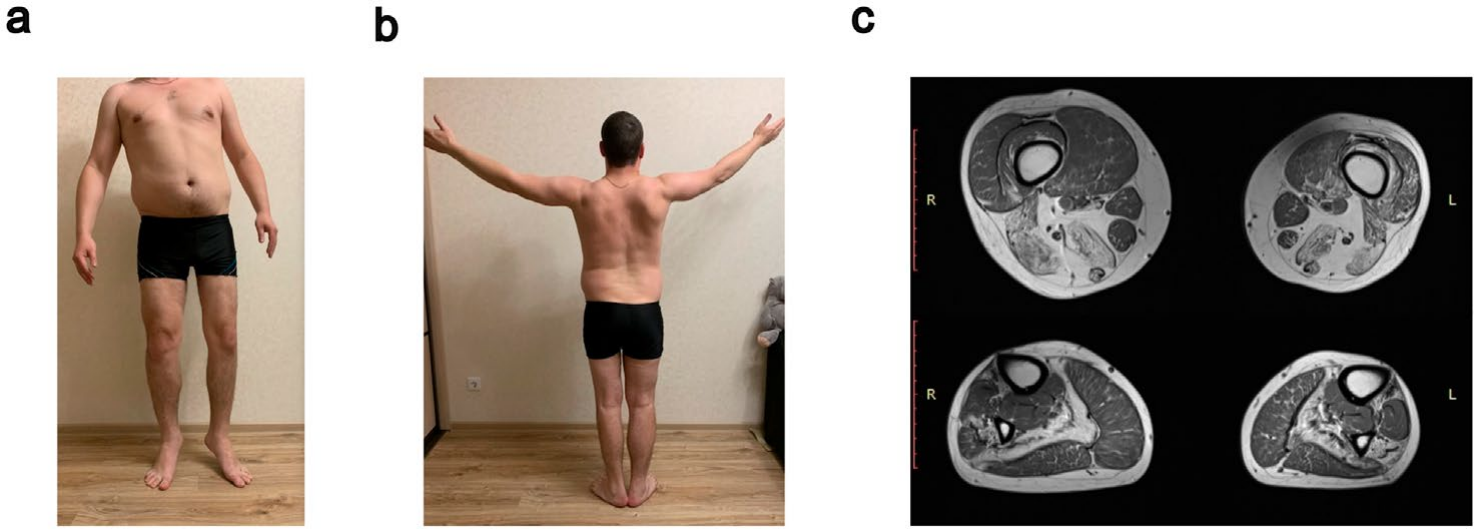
Main clinical findings in Patient 3. (a) Asymmetric lower limb atrophy, left > right. (b) Mild asymmetric scapular winging. (c) Muscle MRI of the lower limbs showing fatty degeneration of hamstrings and adductor magnus and hypotrophy of the left quadriceps on the thigh. And the typical changes in juvenile onset recessive distal titinopathy with severe fatty replacement in soleus and tibialis anterior muscles (in this case, also asymmetric peroneus involvement).
